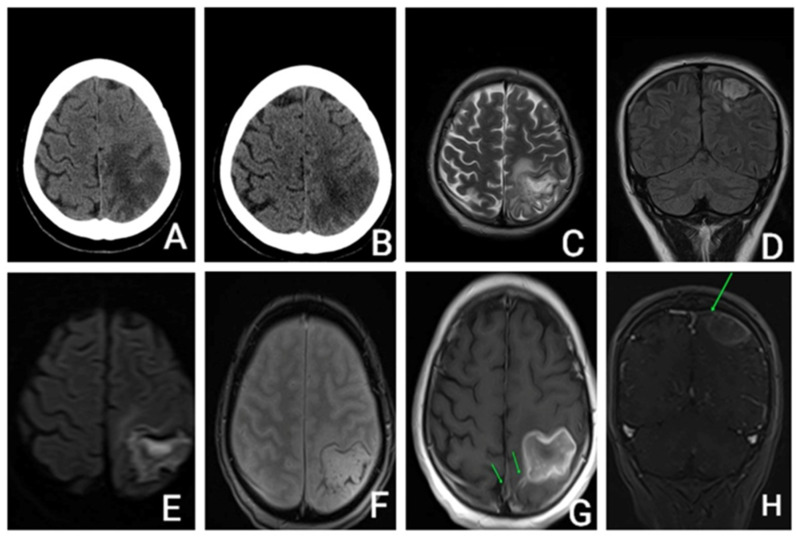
(A,B)—Unenhanced CT axial: (A)—acute parietal stroke with hemorrhagic transformation; (B)—9 days apart—Unenhanced head CT axial: subacute parietal stroke with hemorrhage resorption. (C–H): Brain MRI with and without contrast enhancement: (C)—T2 axial, (D)—FLAIR coronal, (E)—DWI axial, (F)—T2* hemo axial, (G)—T1 axial after i.v. contrast, (H)—T1 coronal after i.v. contrast. Ischemic area with hemorrhage transformation, hemosiderinic deposits mostly in periphery, central restricted diffusion and peripheral enhancement, filling defects of the sagittal venous sinus and of a cortical vein towards the affected left parietal area. CT—computer tomography; T1—weighed image; T2 weighted image; FLAIR—fluid attenuated inversion recovery; DWI—diffusion weighted imaging; T2* hemo—T2 weighted sequence.
